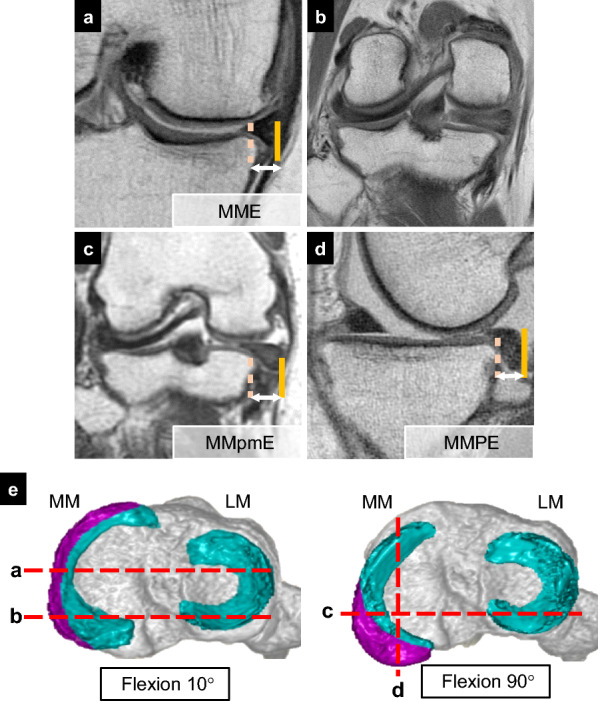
Measurements and reference lines in three-dimensional (3D)-reconstructed MRI of the right knee. MRI images at 10° (a, b) and 90° (c, d) knee flexion. A 3D-reconstructed medial meniscus (MM) from above the tibial plateau with the purple area representing the protruding portion from the tibia (e). a. Medial meniscus extrusion (MME) measured from the tibial edge (dashed line) to the outer edge (line) of the meniscus in the midcoronal plane, excluding osteophytes. b. Root tear gap was sometimes not obvious. c. Medial meniscus posteromedial extrusion (MMpmE) measured from the tibial edge (dashed line) to the outer edge of the meniscus (line), approximately 4 mm anterior to the posterior edge of the tibial plateau. d. Medial meniscus posterior extrusion (MMPE) measured from the tibial edge (dashed line) to the outer edge of the meniscus (line). e. Reference lines for a–d. *MRI* magnetic resonance imaging
